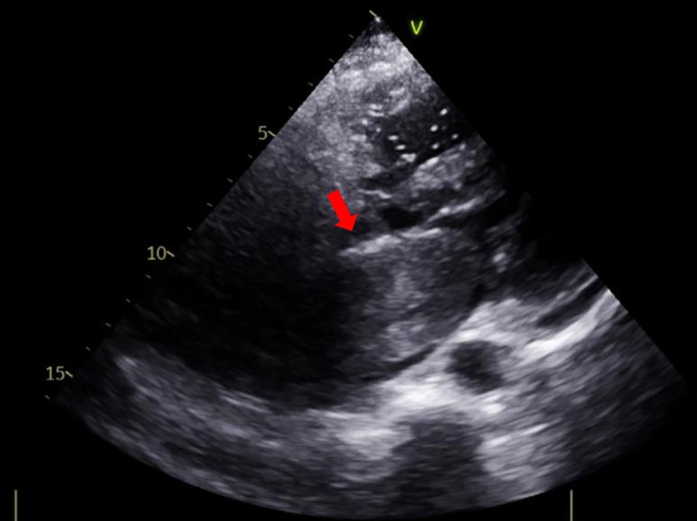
Baseline Echocardiogram Initial echocardiogram revealed a left atrial mass attached to the interatrial septum, protruding into the left ventricle (red arrow).
